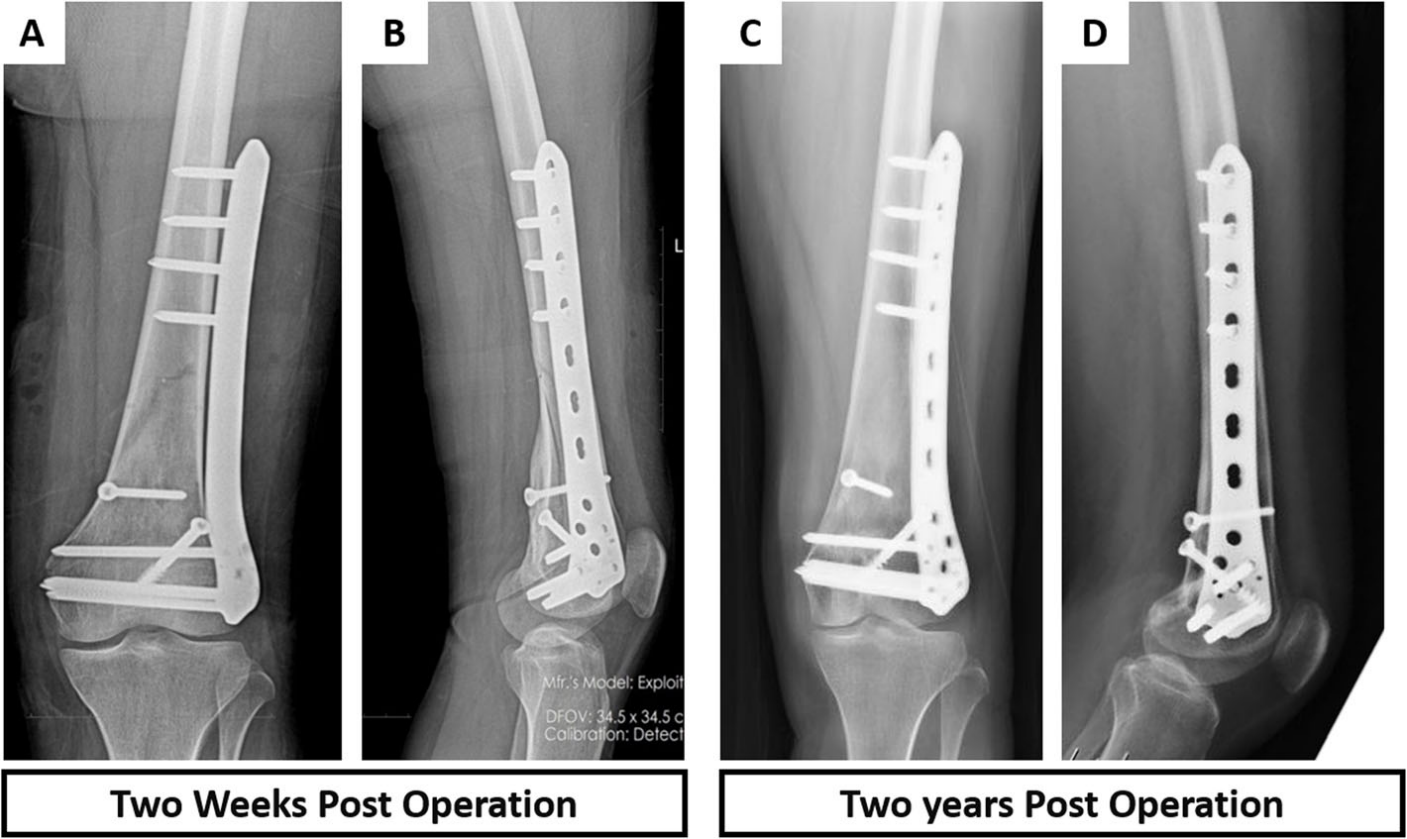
Follow-up images of the representative patient. a, b X-ray images 2 weeks post-operation showing that the gap was clear between the bone block and the host bone. c, d X-ray images 24 months post-operation
